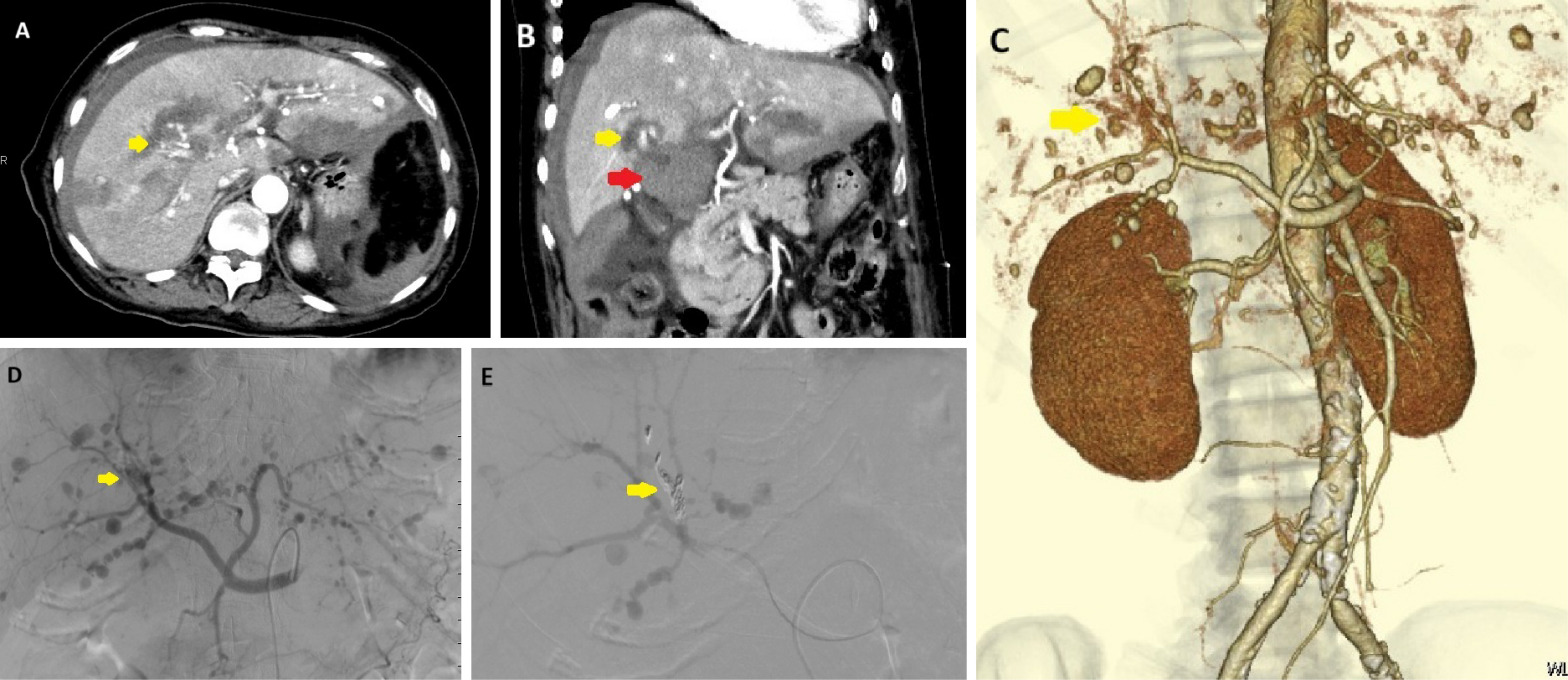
Contrast-enhanced CT and angiography at symptom onset. Contrast-enhanced CT obtained immediately after the onset of abdominal pain shows multiple hepatic artery aneurysms. (A) Axial arterial-phase image and (B) coronal arterial-phase image demonstrate several aneurysms. Intraperitoneal hemorrhage originating from the A5 branch is suspected (yellow arrow), and a hematoma is present in the hepatic hilum (red arrow). No arterial aneurysms were identified in locations other than the liver. (C) Three-dimensional reconstructed CT highlights the extravasation at the A5 branch (yellow arrow). (D) Angiography confirms contrast extravasation from the same site (yellow arrow). (E) Coil embolization was performed at the A5 branch (yellow arrow), clearly showing coil deployment at the bleeding site. CT: computed tomography.
